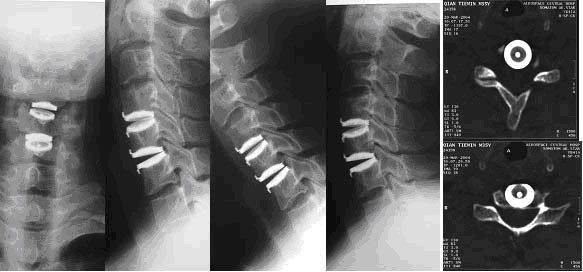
24 months postoperative anteroposterior and lateral x-rays after disc replaced at C4/5,5/6, Lateral flexion/extension x-rays show restored normal ROM. CT scan show no prosthesis excusion or heteostification.
